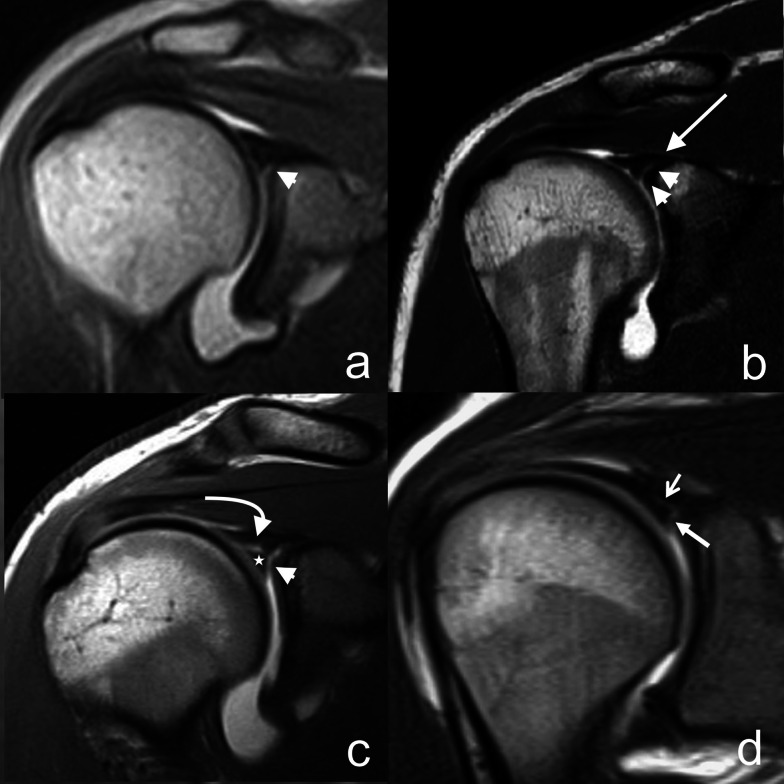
The images in a–d are all T1-weighted MR arthrographies, acquired in coronal-oblique section after intraarticular injection of approximately 20 ml of paramagnetic contrast agent. In particular, image a shows a type I SLAP lesion (arrowhead); image b shows a type II SLAP lesion (arrowheads) that does not reach the bicipital anchor (arrow); image c displays a type III SLAP lesion where the lesion (arrowhead) extends in a centrifugal direction alongside the bicipital anchor (curved arrow) delimiting a triangular fragment of fibrocartilage (star); image d displays a type IV SLAP lesion where the lesion (thick arrow) reaches and interrupts part of the bicipital anchor (thin arrow). c and d are reprinted with the permission of E. A. Genovese [3]
